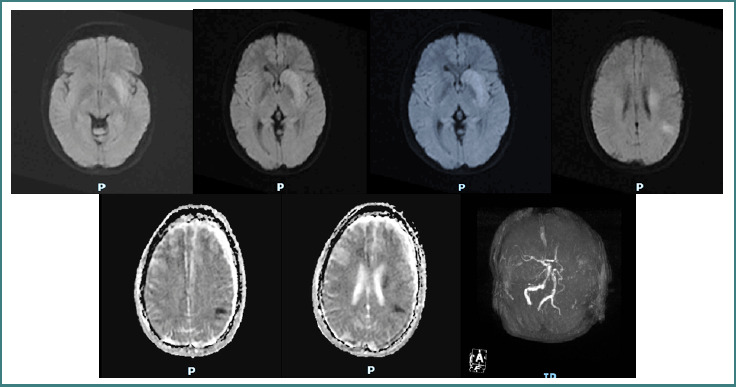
Brain MRI at acute presentation. Axial diffusion-weighted imaging (DWI) sequence demonstrating areas of restricted diffusion in the left basal ganglia and ipsilateral parietal cortex (Wernicke area), consistent with acute ischemic lesions.
